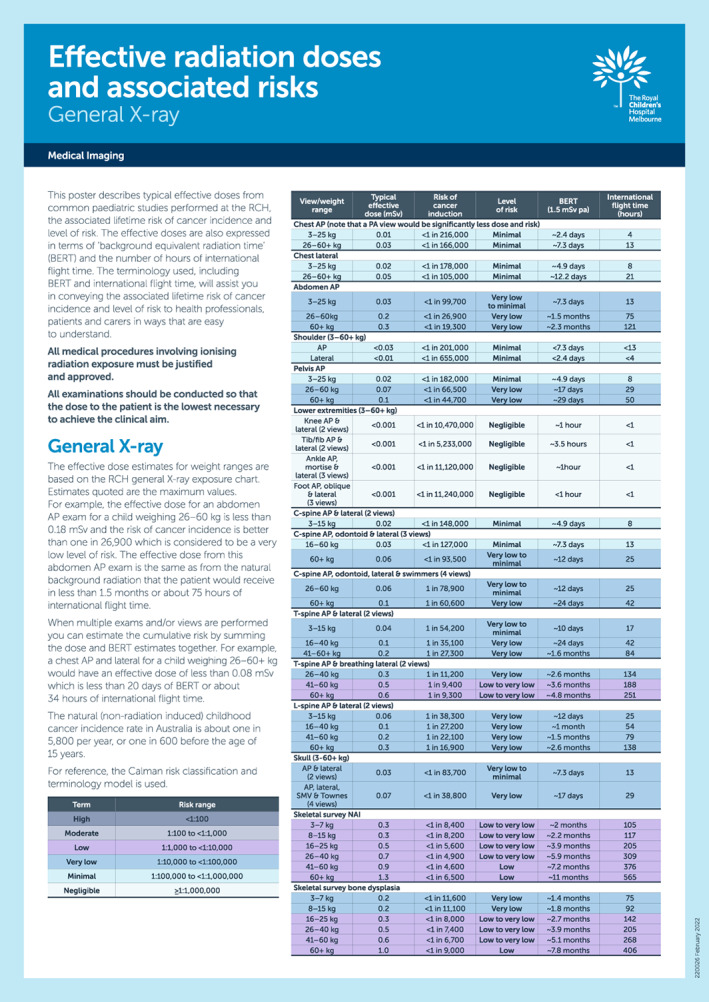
Typical effective doses, risk of cancer incidence, level of risk, comparison with natural background equivalent radiation time (BERT) of 1.5 mSv per annum and comparison with international flight time for commonly performed for general radiography examinations at the RCH.
